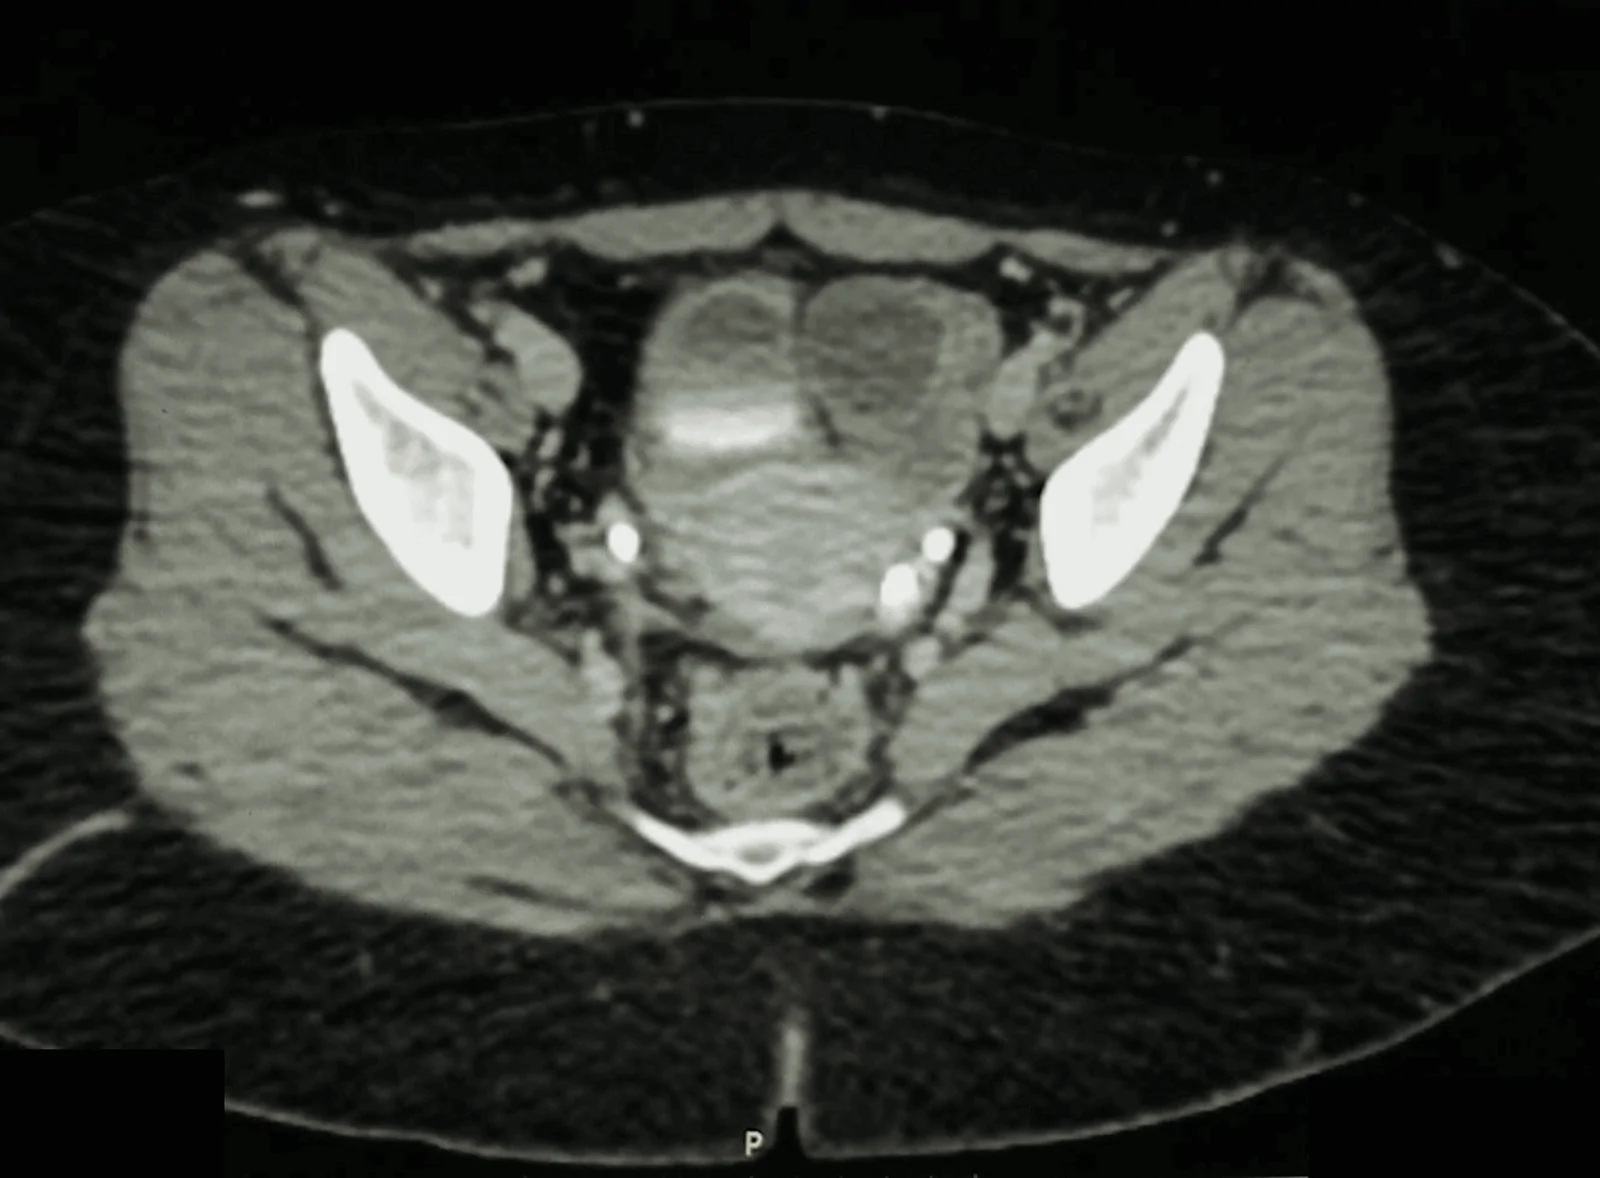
Axial contrast-enhanced computed tomography (CT) image of the pelvis showing the left adnexal cystic lesion (arrow) with adjacent ill-defined calcific foci in the left adnexal region. No significant abnormalities are identified within the visualised bowel loops or ileocaecal region.
